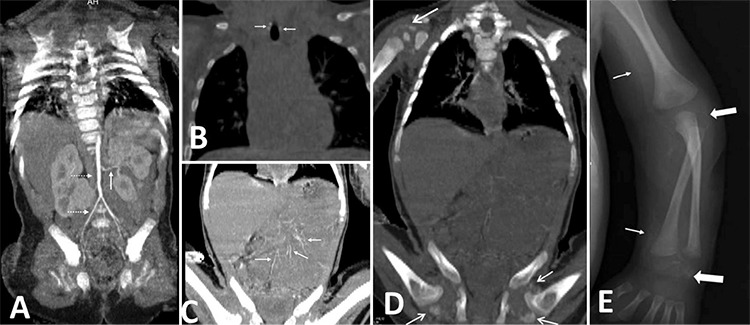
At age three months, coronal non-contrast computed tomography of abdomen and chest, A) Diffuse narrowing is seen in bilateral renal arteries, abdominal aorta and bilateral iliac arteries, B) At the level of the larynx, soft tissue calcifications are observed in the paratracheal region and around the hyoid bone, C) There are linear hyperdensities consistent with calcification in the mesenteric artery and branches, D) Periarticular calcification in the right shoulder joint and in the right hip joint, E) At age three months, on baseline radiograph; the left wrist shows arterial calcification of the brachial and radial arteries (thin arrow) and intra- and peri-articular calcifications in left elbow and wrist joints (thick arrow)
